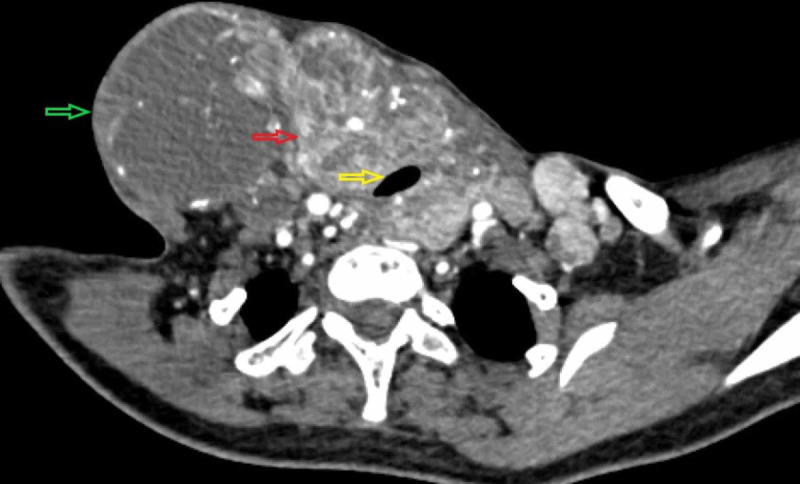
Preoperative axial CT scan section showing massive lymphadenopathy (green arrow) associated with a hypervascular thyroid mass (red arrow) showing microcalcifications, causing narrowing of the trachea (yellow arrow).
